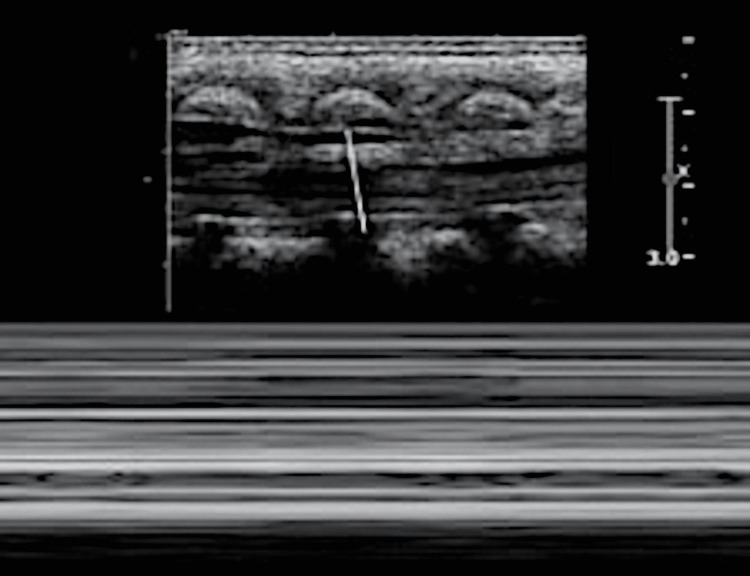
Spinal ultrasound M-mode captured decreased dependent motion of the cauda equina. Radiological images obtained by the authors.
